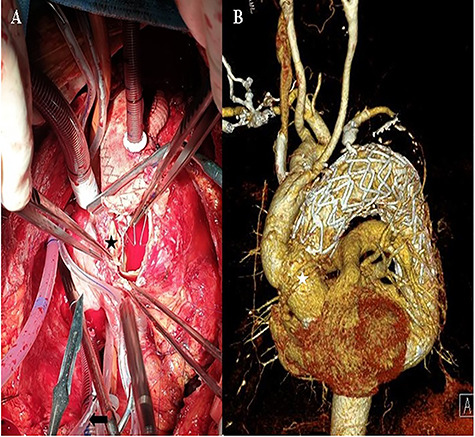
A: Intraoperative view of the transected ascending aorta due to the thoracic stent graft (black asterisk). B: The post-operation computed tomography image shows the replaced ascending aorta (white asterisk).
